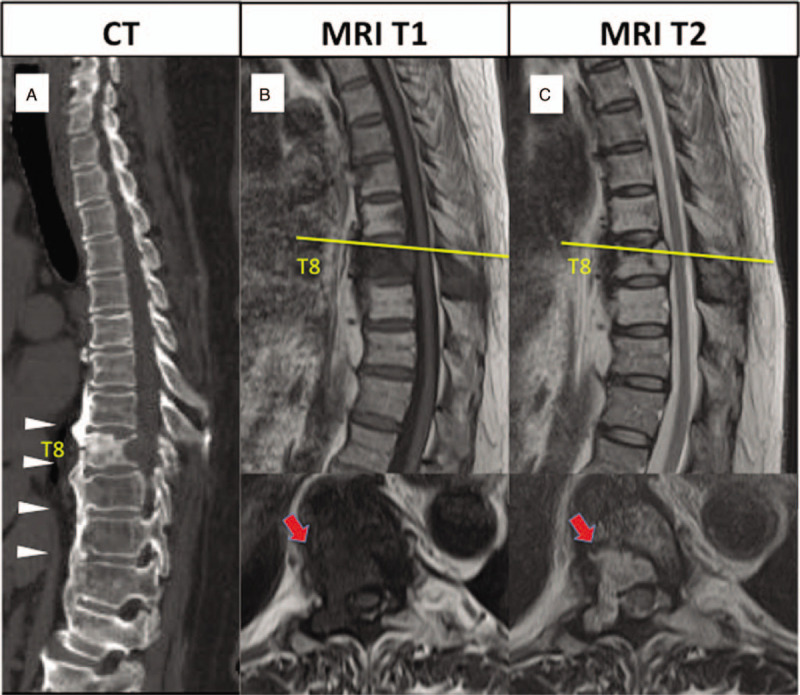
(A) Computed tomography scan reveals fusion of vertebra from T5 to T12 because of ossification of the anterior longitudinal ligament (white arrow). (B) T1-weighted and (C) T2-weighted MRI scans show spinal Metastasis at the T8 vertebra and the right pedicle with no stenosis.
